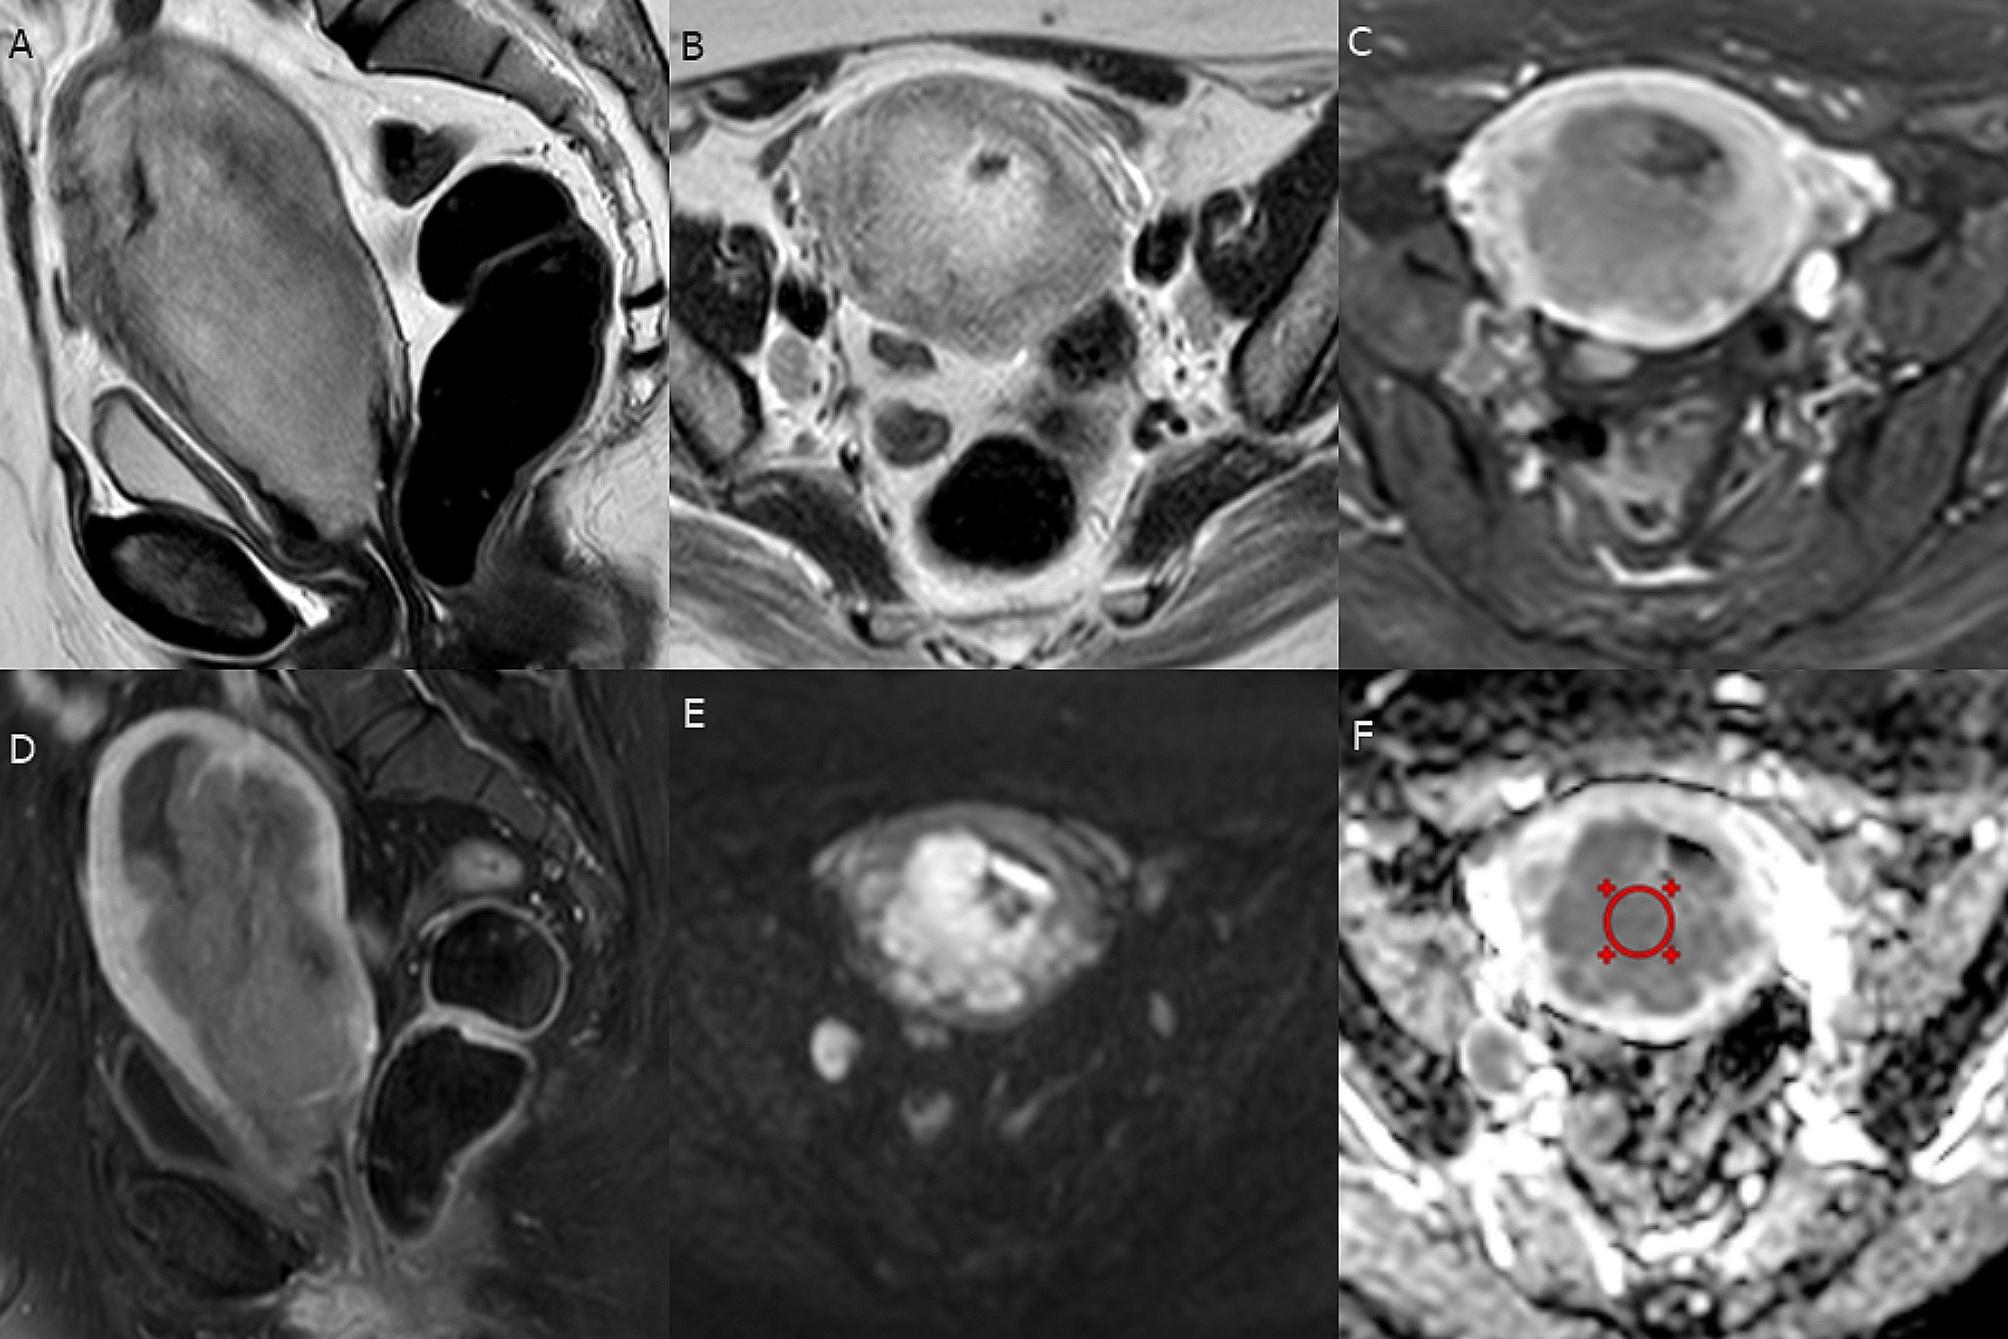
A patient with endometrial serous carcinoma and LVSI (FIGO stage III C1). (A and B) Sagittal and axial oblique T2-WI respectively showed the large endometrial mass of heterogeneous intermediate signal intensity with lost hypointense junctional zone and deep MI, interrupted hypointense cervical stroma denoting CSI, and globular suspicious right internal iliac LN. (C & D) Axial oblique and sagittal contrast-enhanced T1-WI revealed a heterogeneous enhancement of the endometrial mass, deep MI, CSI, and heterogeneously enhanced necrotic right internal iliac LN. (E & F) Axial DWI at b = 1000 and ADC map respectively revealed marked diffusion restriction of the endometrial mass and right iliac LN with ADCmean value = 0.77 × 10 –3 mm/sec
